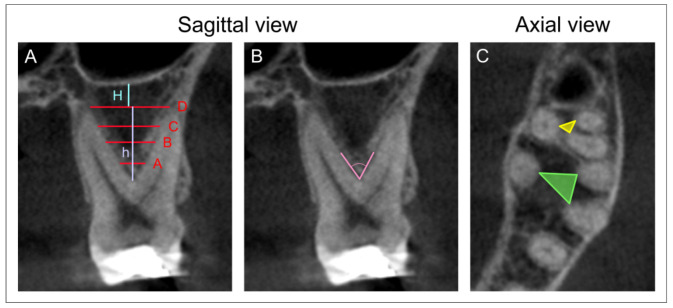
CBCT scans and landmarks of interest. (A)—Sagittal view: the interradicular septum width at level A (at 2 mm distance from furcation), B (at the mid length of h), C (at 2 mm distance from the interradicular septum base), and D (at the interradicular septum base); the interradicular septum height—h; the distance between the interradicular septum base and the sinus floor—H. (B)—Sagittal view: the interradicular furcation angle. (C)—Axial view: the interradicular septum surface perimeter and the surface area (for the first molars—green, and the second molars—yellow line).
